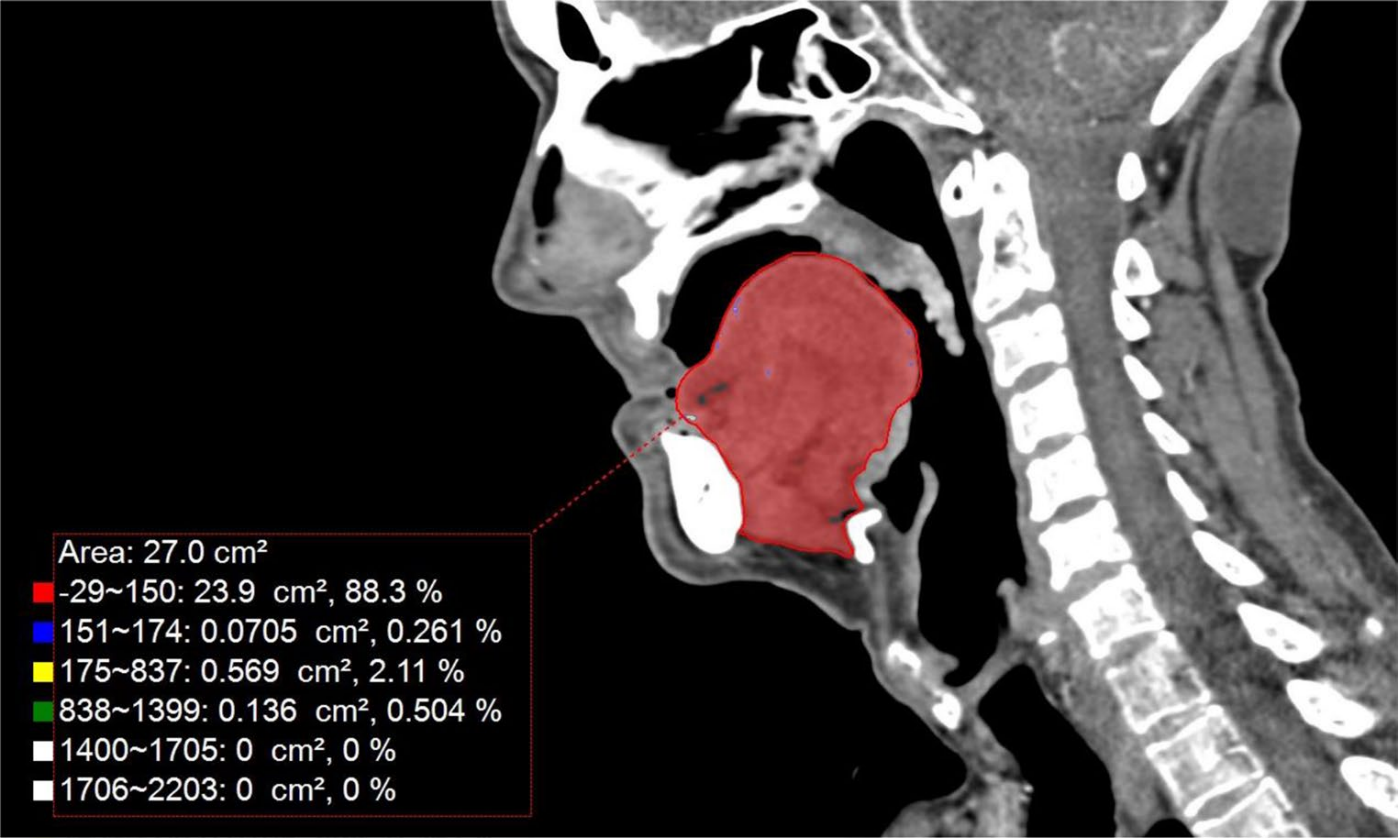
Example of the tongue complex muscles area measurement on a mid-sagittal CT slice. The red area represents the delineated muscles. The upper value (area: 27.0 cm^2^) corresponds to the sagittal area of the tongue complex muscle. Other values with color squares correspond to measurements with Hounsfield unit threshold tool for different muscle densities. Note, that lymphoid tissue was excluded from the delineation at the base of the tongue
